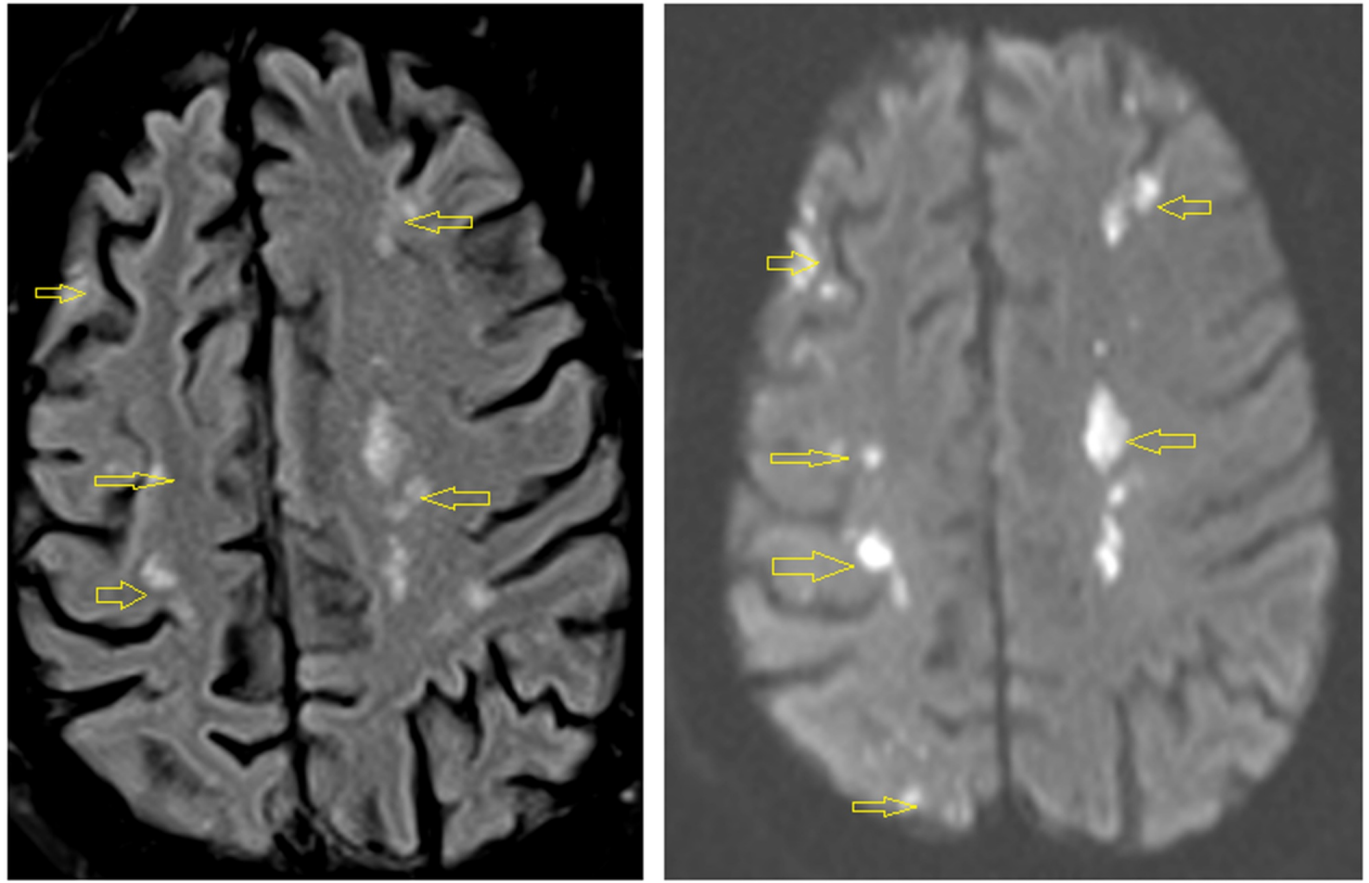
Brain MRI Multiple areas of restricted diffusion throughout the hemispheres and posterior fossa in multiple vascular distributions, suggestive of multiple emboli with acute/subacute infarctions (yellow arrows).
